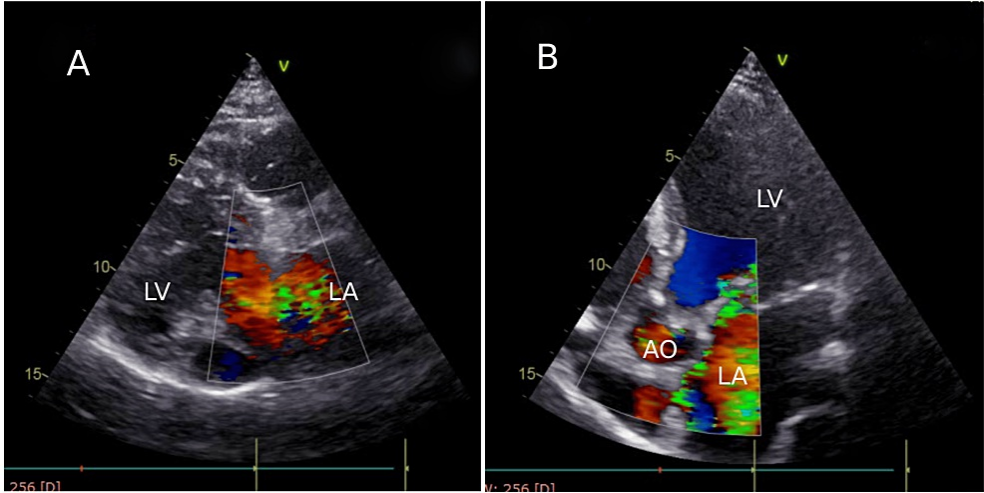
Transthoracic echocardiogram with color Doppler imaging A: Parasternal long-axis view with color Doppler showing a rapid jet of blood backflow in the LA, suggesting severe MR. B: Apical five-chamber view with color Doppler showing rapid, turbulent blood backflow from the LV into the LA, confirming severe MR. LV: left ventricle, LA: left atrium, AO: aorta
